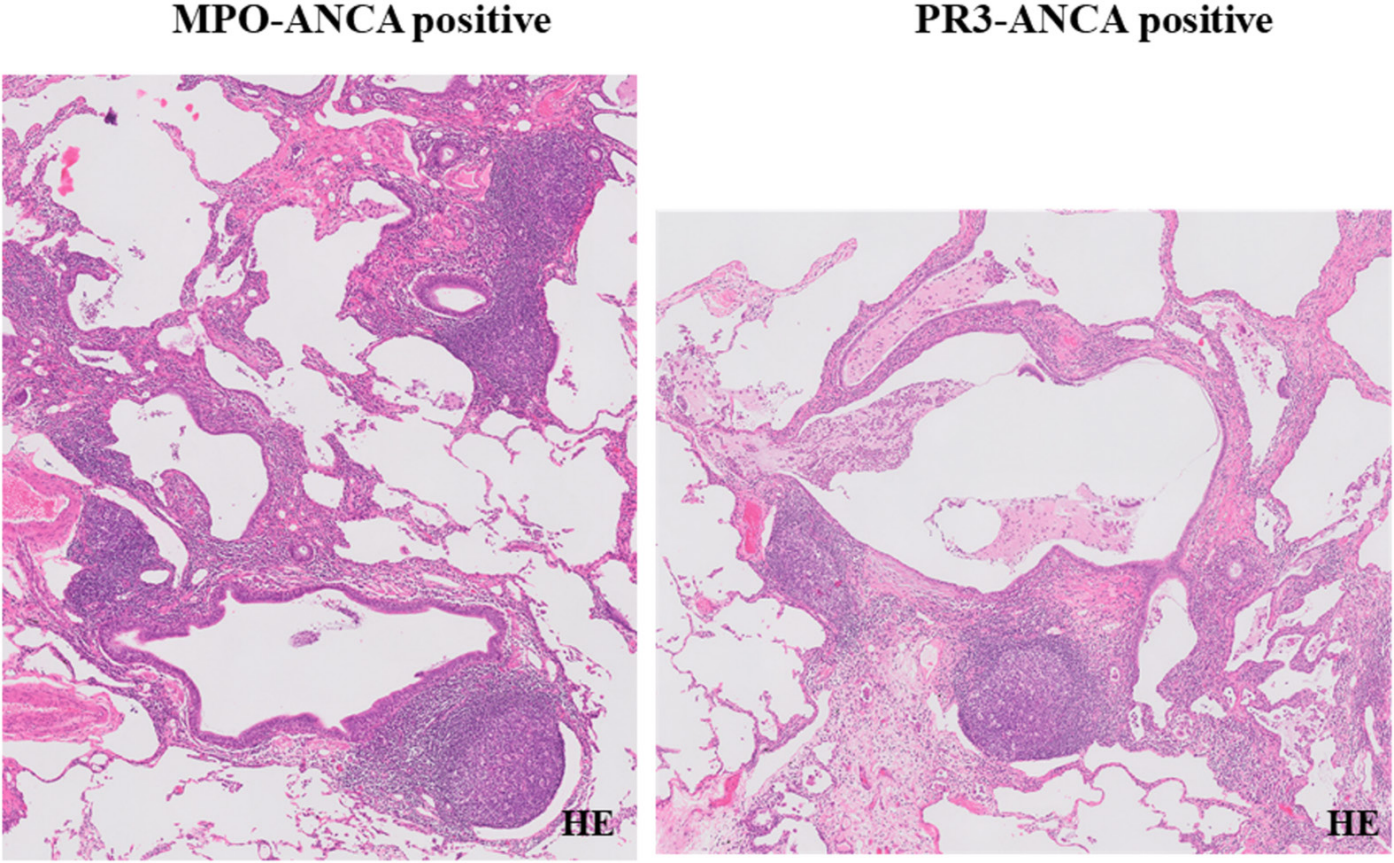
Pathological findings of nodular lesions on HRCT image showing lymphocytic infiltration and lymphoid follicles with germinal center around bronchiolar walls.
